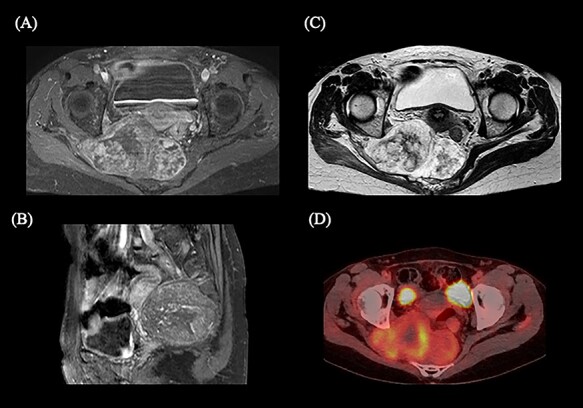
Magnetic resonance imaging (MRI) and 2-deoxy-2-[^18^F]fluoro-d-glucose (FDG)-positron emission tomography (PET)/computed tomography (CT) showing the sacral chordoma before spacer placement and the initiation of carbon ion radiotherapy. (A) Axial MRI of the gadolinium-enhanced T1-weighted image. (B) Sagittal MRI of the gadolinium-enhanced T1-weighted image. (C) Axial MRI of the T2-weighted axial image. (D) Axial FDG-PET/CT.
